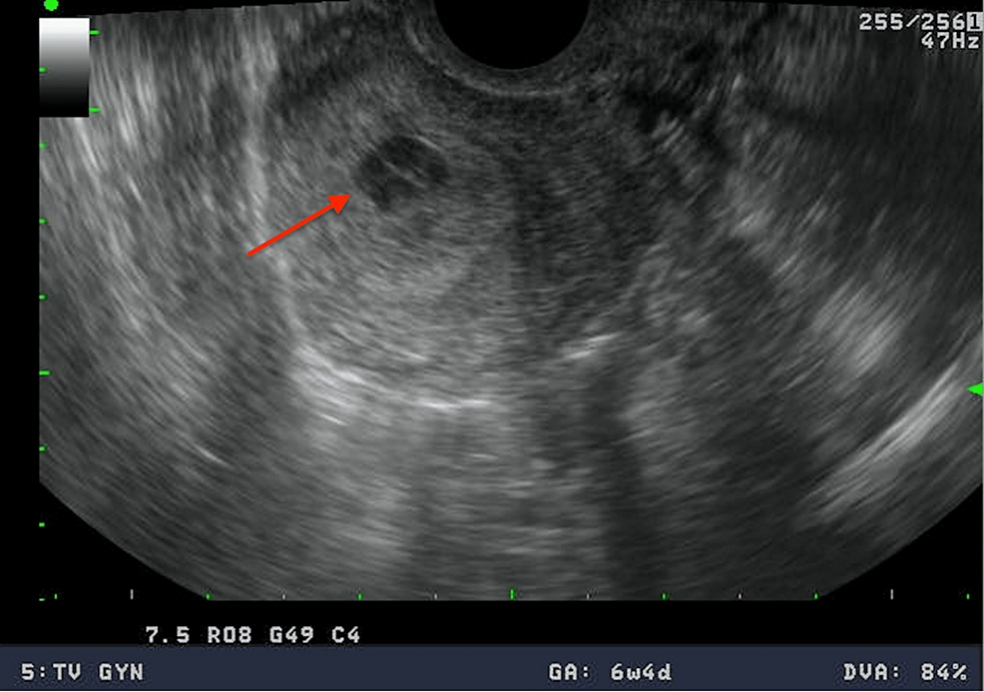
Transvaginal ultrasound demonstrating a possible intrauterine gestational sac following clomiphene administration
